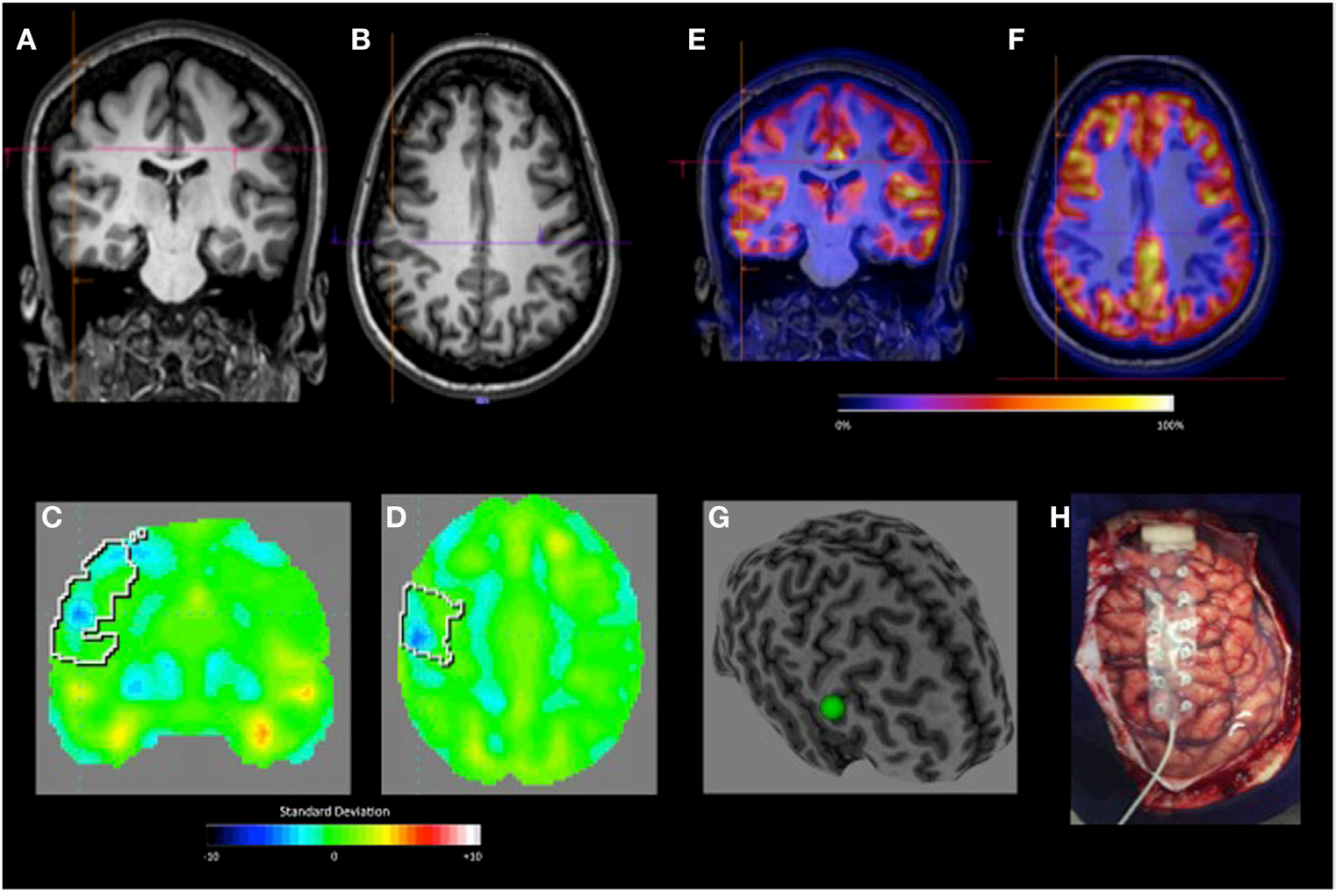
Gliosis (oligodendrogial hyperplasia). (A,B) Coronal and axial negative-MRI. The visual-positron emission tomography (PET) was initially considered normal. (C,D) Quantitative-PET showing hypometabolic area in the right central area with −4.8 SD. (E,F) Coronal and axial PET/MRI co-registration. (G) Curvilinear reconstruction show discrete convergence of sulci and gyrus. (H) Resected brain tissue.
